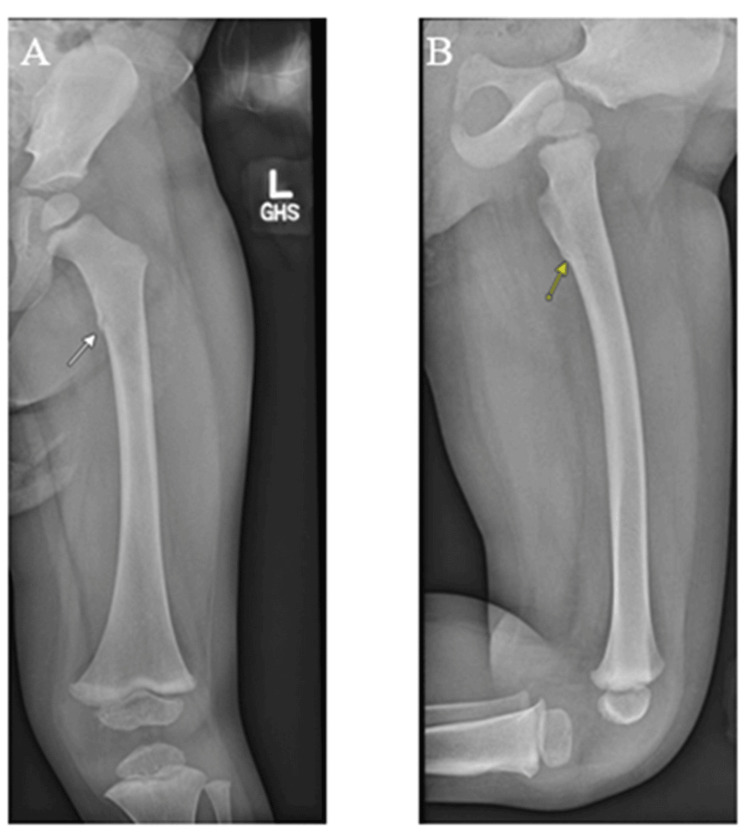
Initial imaging for case 2, including (A) AP and (B) lateral radiographs of the left lower extremity demonstrating a nondisplaced fracture of the medial cortex of the subtrochanteric proximal femur
